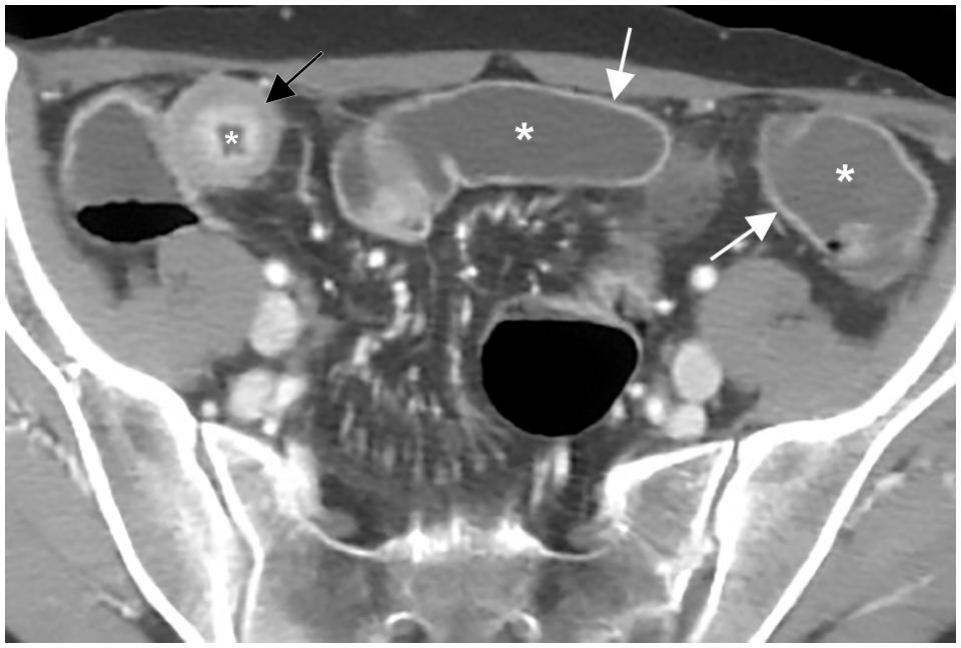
CT-enterography in a patient with known Crohn’s disease. The examination, performed after iodinated contrast medium injection, shows some ileal loops (white asterisks) distended from the oral neutral contrast agent (polyetilen-glycole solution). Hypodensity of the bowel lumen allows better visualization of the intestinal wall and mural enhancement characteristics (white arrows). Marked thickening and stratified contrast enhancement of an ileal loop in the right lower abdominal quadrant are also evident (black arrow).
